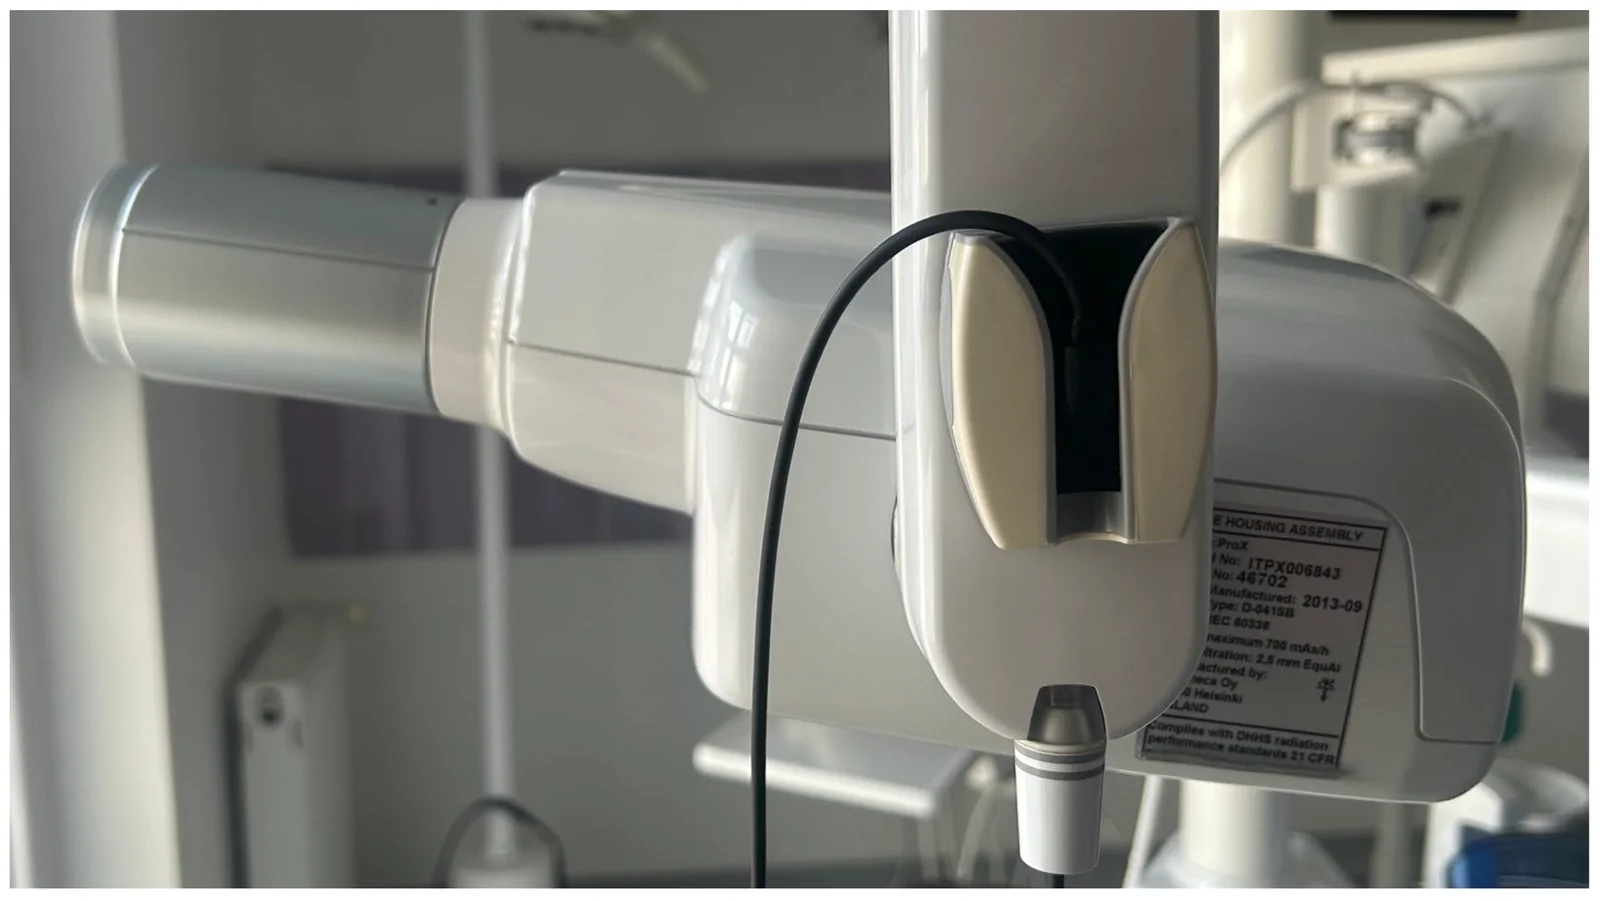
Planmeca ProX X-ray unit from the dental clinic.
